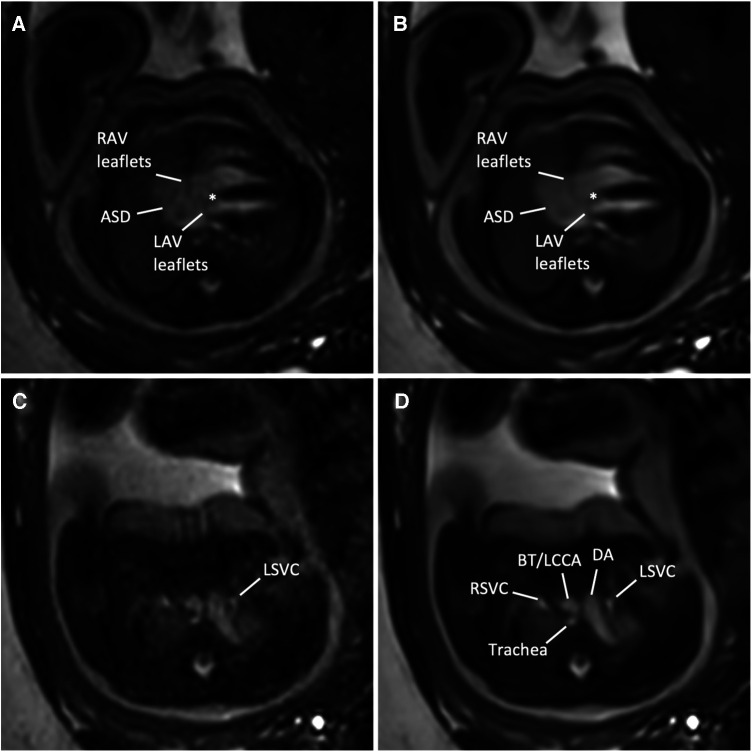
(A,C) Standard CS and corresponding (B,D) DL image reconstructions of an axial balanced steady-state free precession cine sequence in a 35-year-old woman at a gestational age of 36 weeks and 3 days with an intermediate atrioventricular (AV) septal defect of the fetus. Images at AV level (A,B) revealed a large (type I and II) atrial septal defect (ASD), right AV and left AV leaflets, and a small defect in the basal portion of the interventricular septum (asterisk), indicating an intermediate-type AV canal. Note the clear delineation of the AV leaflets in the DL denoised image (B) compared with the standard CS reconstructed image (A) In addition, MRI revealed a left persistent superior vena cava (LSVC, C,D), draining into the coronary sinus (not shown). Adjacent structures at this level are the right superior vena cava, the ductus arteriosus, the supra-aortic vessels, including the brachiocephalic trunk (BT) and the left common carotid artery (LCCA), and the trachea. Both standard CS (Supplementary Movie S3) and DL-reconstructed cine images at the AV level (Supplementary Movie S4) are also provided. DA, ductus arteriosus; LAV, left atrioventricular valve orifice; RAV, right atrioventricular valve; RSVC, right superior vena cava.
